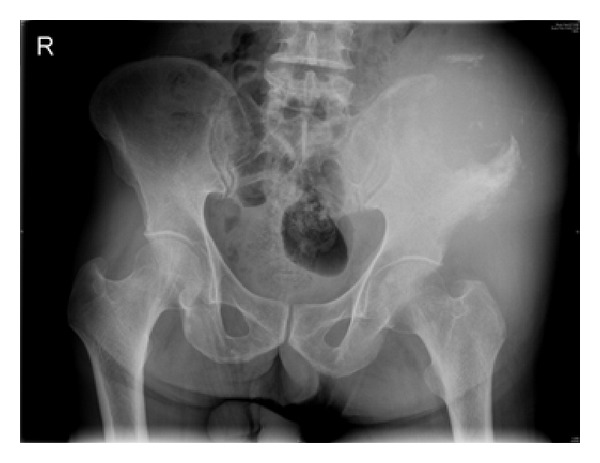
Plain radiograph of pelvis showing a permeative lesion in the left ilium with significant cortical destruction.
